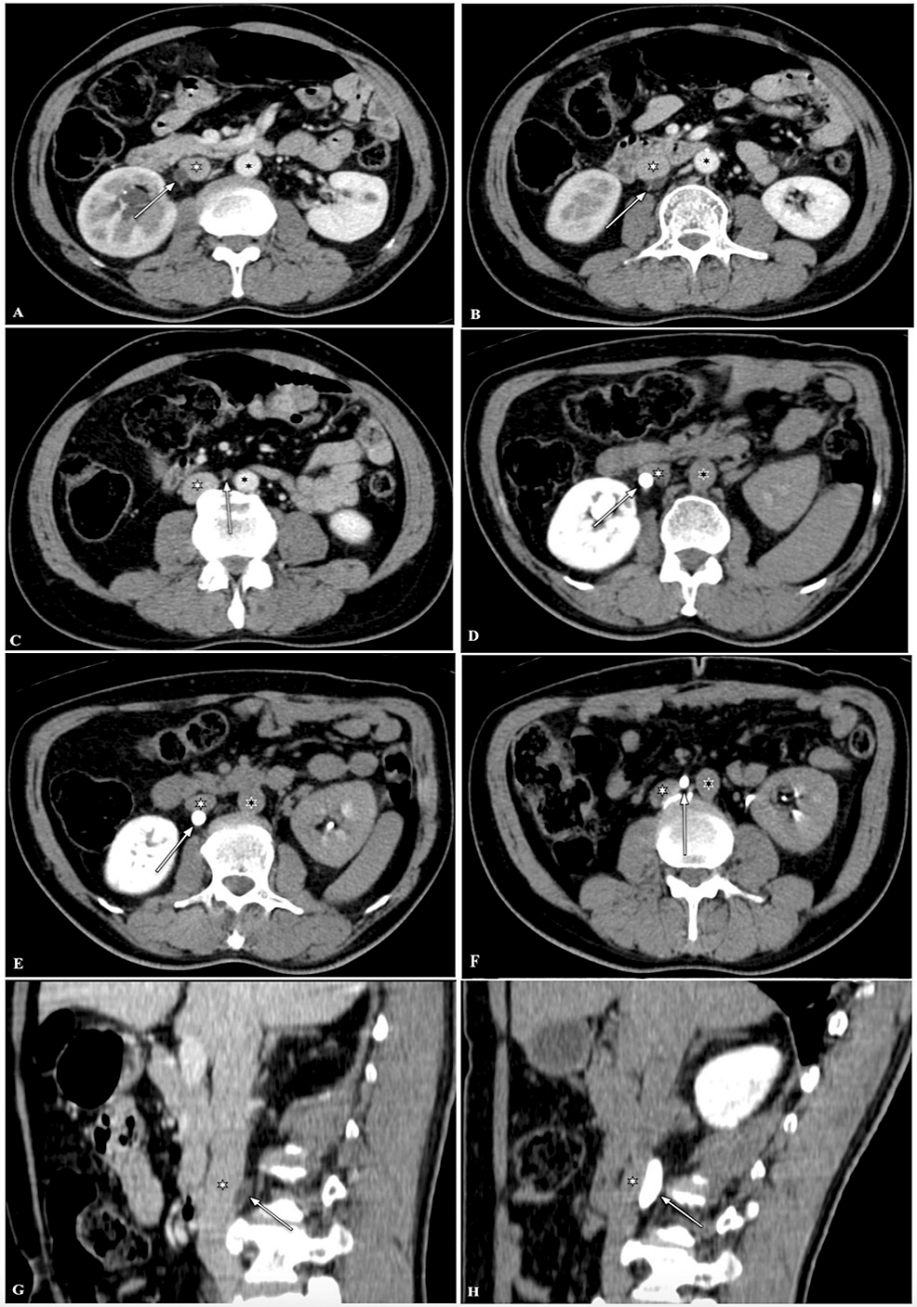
Computed tomography urography images (A-C) Axial sections in venous phase), (D-F) Axial sections in delayed phase, and (G, H) Sagittal sections in venous and delayed phases) shows the right mid ureter coursing medially (paramedian to the inferior vena cava, IVC), posterior to the IVC, and in-between the IVC and abdominal aorta (at the level of the L2-L3 vertebrae, L3 vertebra, L3-L4 vertebrae, respectively) causing mild proximal hydroureteronephrosis. Note: Right mid ureter (large white arrow), IVC (small white asterisk), and abdominal aorta (small black asterisk).
